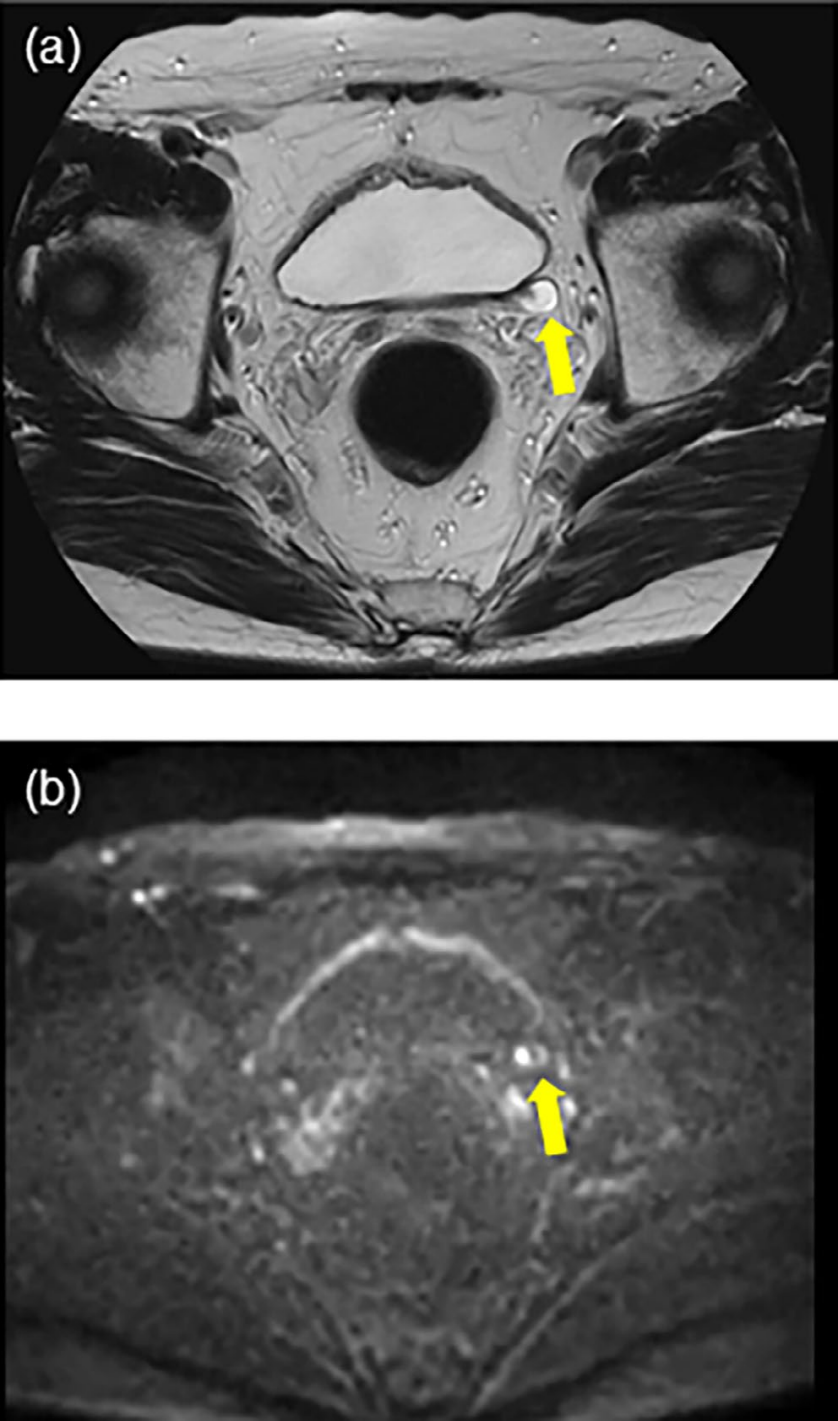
MRI showing bladder tumor in an isolated diverticulum (yellow arrows). (a) T2‐weighted image. (b) Diffusion‐weighted image.
